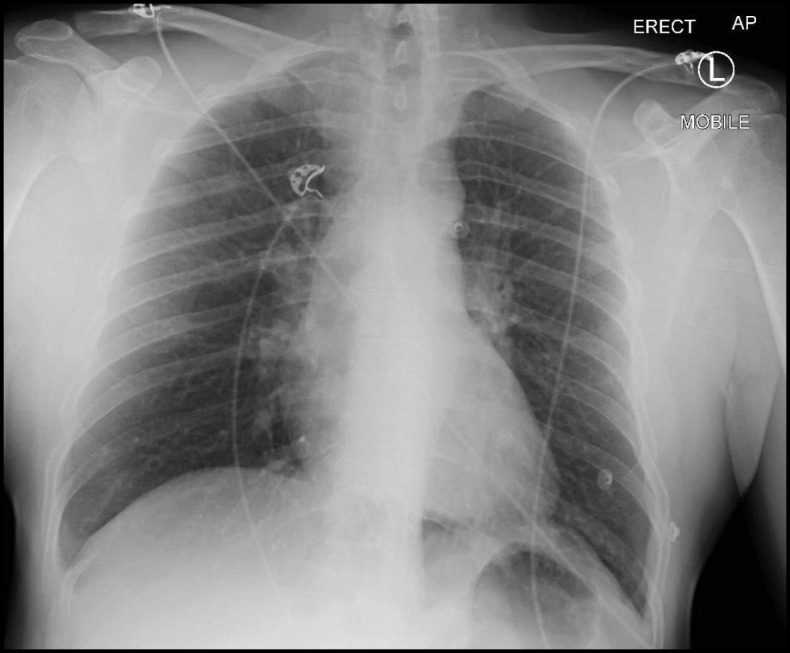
Effectively clear plain erect anteroposterior chest x-ray at presentation
